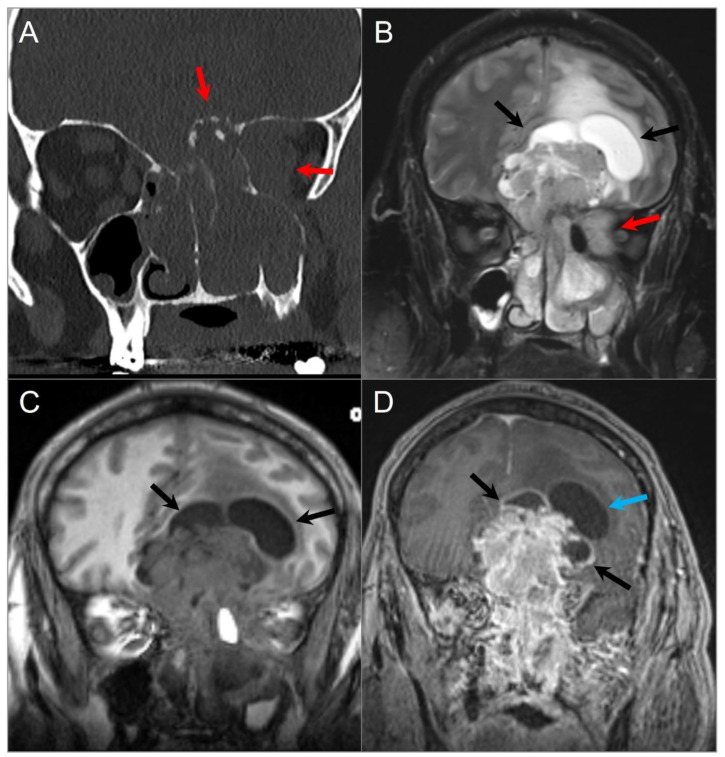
Olfactory neuroblastoma. (A) Coronal CT image shows a soft tissue density lesion centered in the left greater-than-right superior nasal cavity with left intraorbital and anterior cranial fossa extension through erosion of the cribriform plate and lamina papyracea (red arrows). (B) T2W and (C) noncontrast T1W coronal images reveal a dumbbell-shaped mass with heterogeneous signal intensity extending into the superior nasal cavity, paranasal sinuses, anterior cranial fossa, and left orbit (red arrows). The ‘waist’ of the dumbbell is centered at the cribriform plate. The mass causes marked mass effect on the adjacent frontal lobes with vasogenic edema. Peritumoral cysts are also noted at the tumor–brain interface (black arrows). (D) Coronal postcontrast T1W image shows intense heterogeneous and irregular enhancement of the lesion with smooth wall enhancement of the several peritumoral cysts, while one shows no appreciable enhancement (blue arrow).
